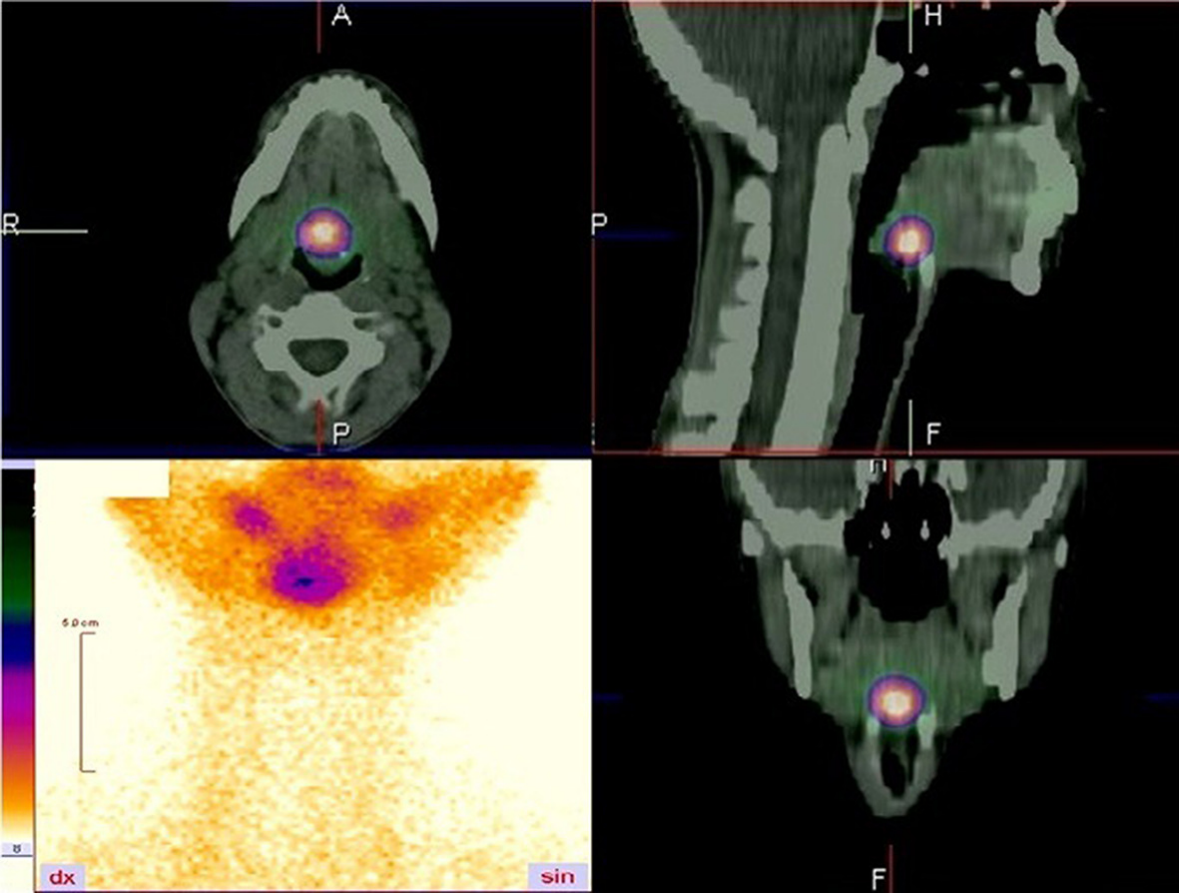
Single photon emission CT/CT using technetium-99m showed radioisotope uptake at the root of the tongue (in the same location as the mass seen on CT scan) and no uptake of radioisotope in the normal thyroid location.
